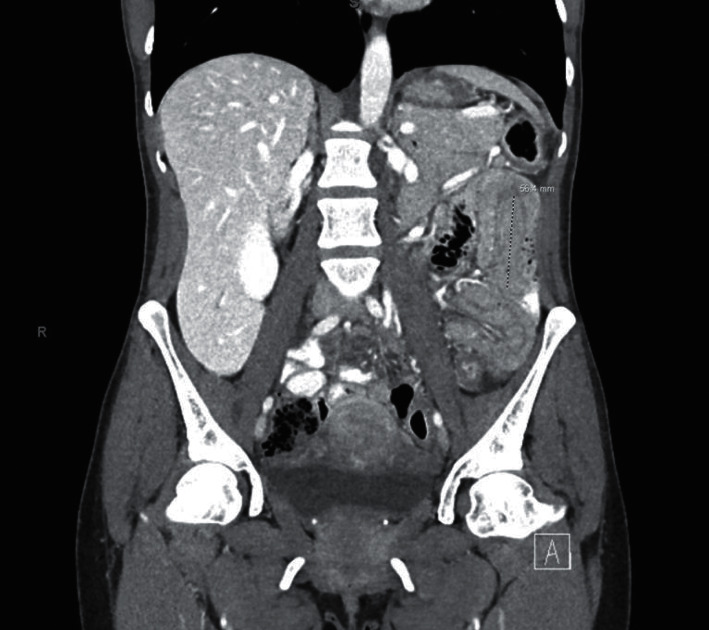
CT scan showing intussusception in the left hemiabdomen.
